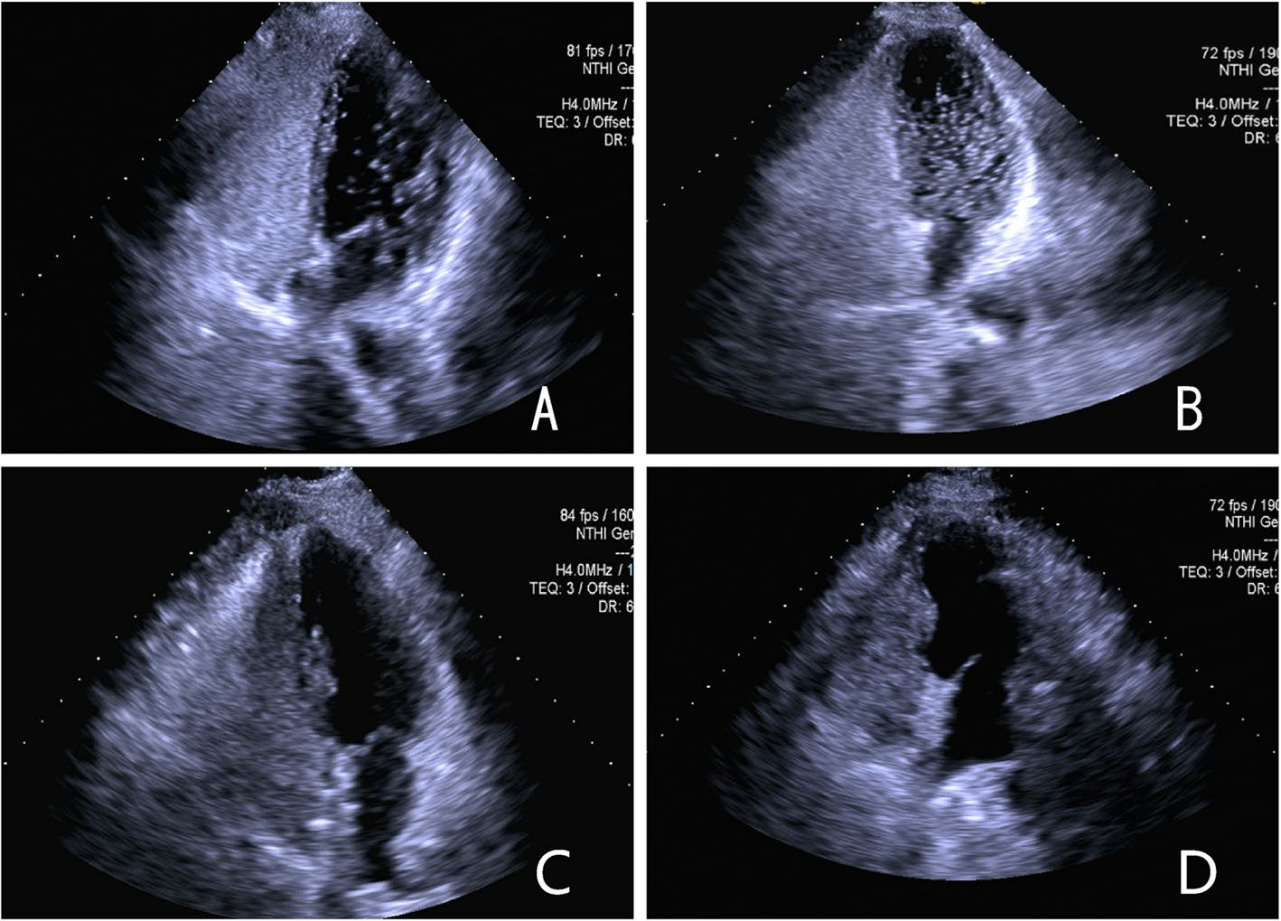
Results of contrast echocardiography of right heart
